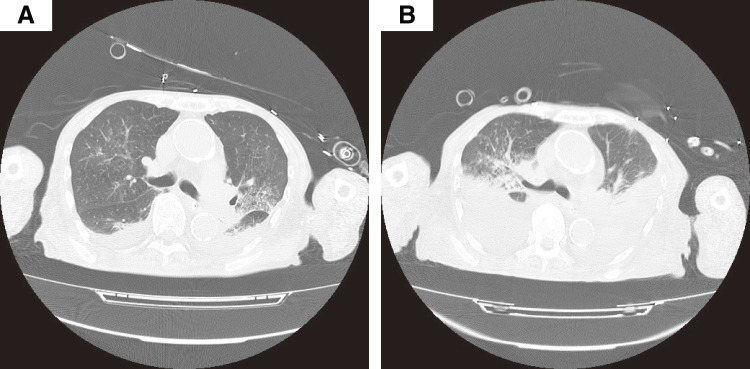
(A) Thoracic CT scan on hospital day 7 at the onset of respiratory failure, demonstrating bilateral ground-glass opacities and moderate pleural effusions. (B) Follow-up CT scan on hospital day 29 demonstrating a marked interval increase in pleural effusions, resulting in extensive compressive atelectasis. CT: computed tomography
